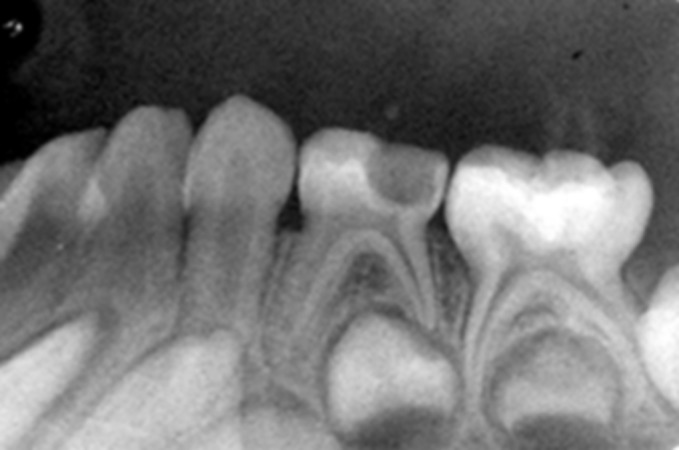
Preoperative IOPA radiograph of hydroxyapatite
